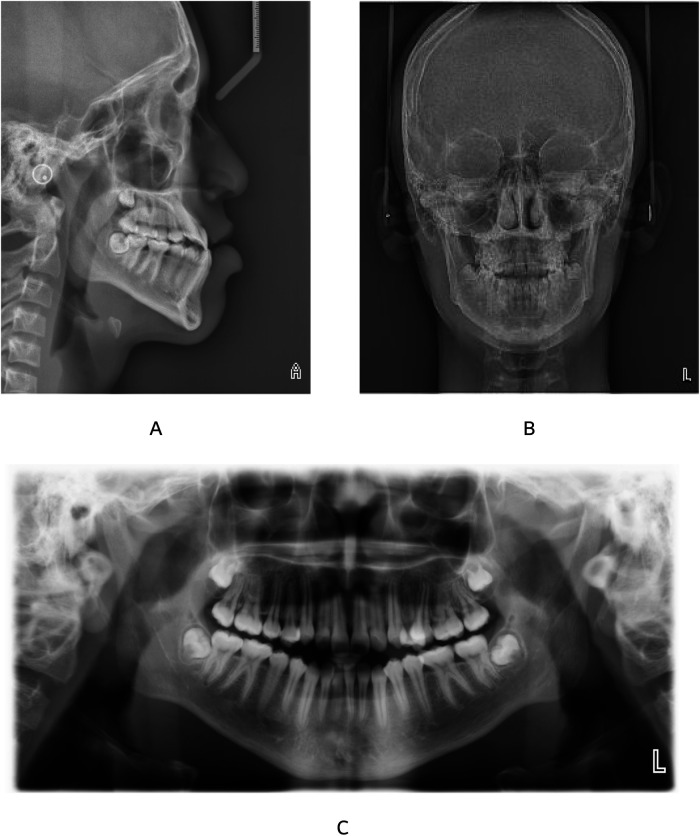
Pretreatment radiographs. Lateral cephalogram (A) and posteroanterior (PA) cephalogram (B) obtained pretreatment. Panoramic radiograph (C) showing the presence of all four third molars without evident pathological findings.
